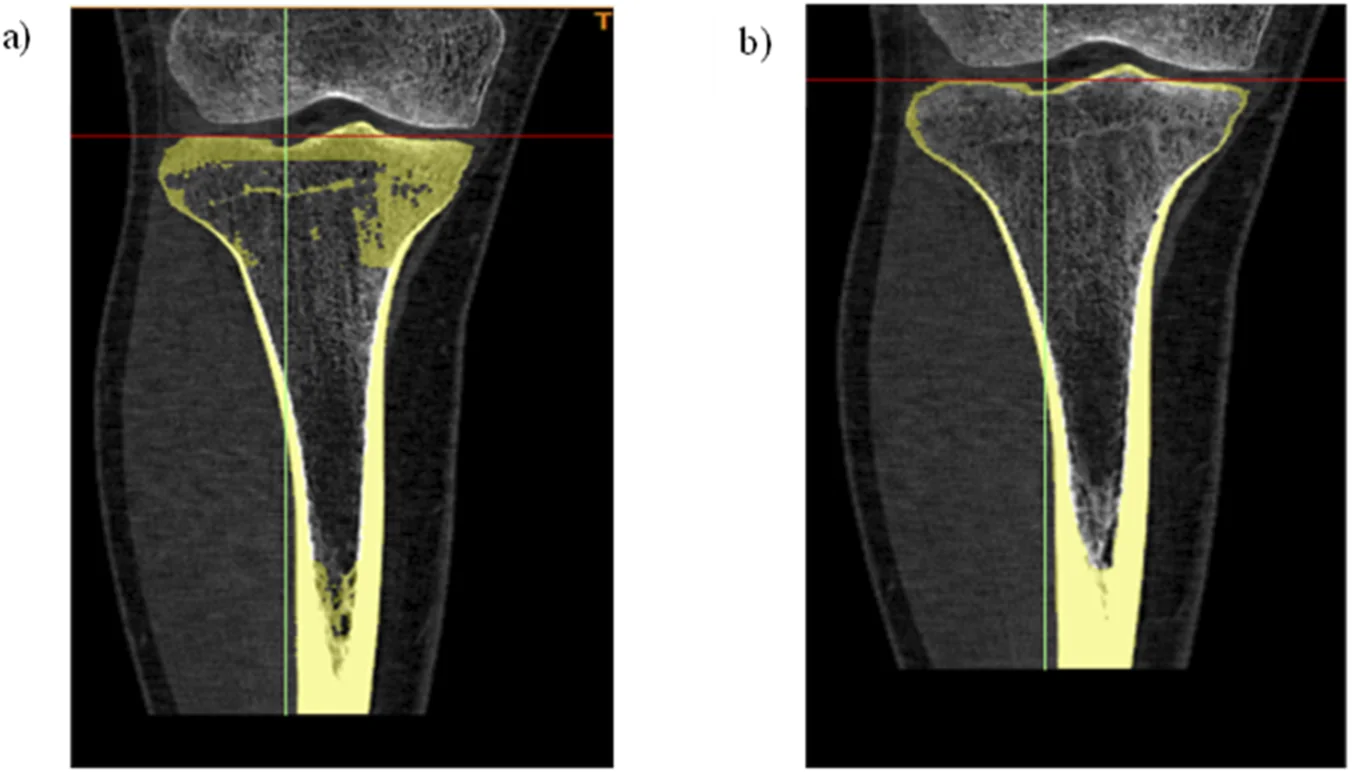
MRI. (a) Image obtained by tomography. (b) Image correction.
